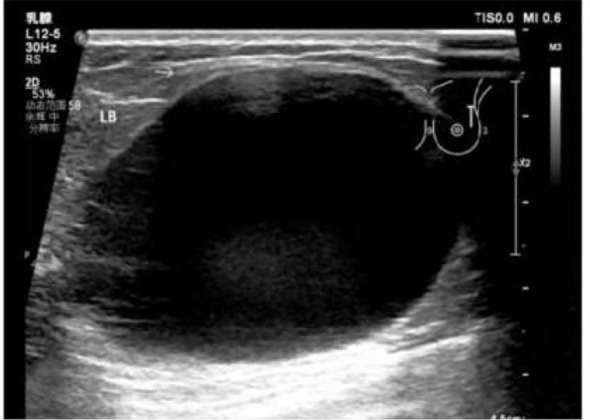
Ultrasound findings of Case 2 (April 2025). Breast ultrasonography identified a 49mm×49mm×33mm well-circumscribed cystic and solid lesion in the upper outer quadrant of the left breast. The lesion demonstrated predominantly hypoechoic echotexture with low-level internal echoes. Color Doppler imaging showed no definite internal vascularity.
